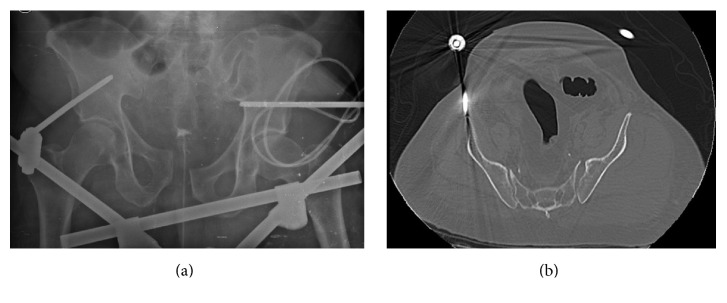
(a) Anteroposterior radiograph of the pelvis showing pubic symphysis diastasis. (b) CT scan axial view confirms pelvic ring injury due to sacroiliac joint subluxation without bone involvement.
